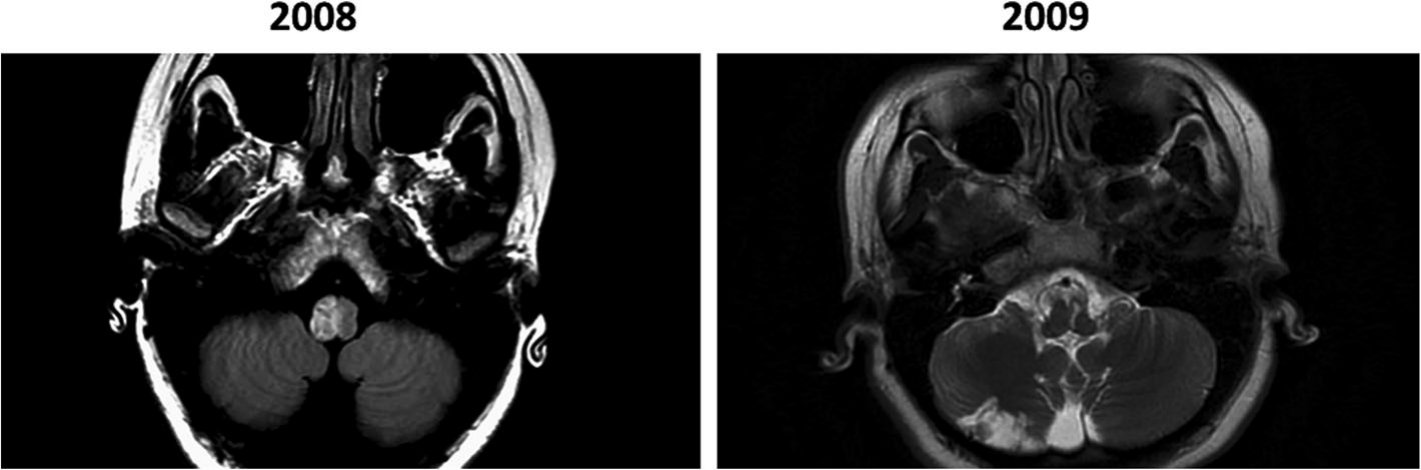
Serial Axial T2 FLAIR MRI. Left panel: increased signal intensity in the right hemi-medulla. Right panel: a head MRI obtained 4 months later revealed a strip of increased signal intensity in the left medial medulla that affects the left medial IO
